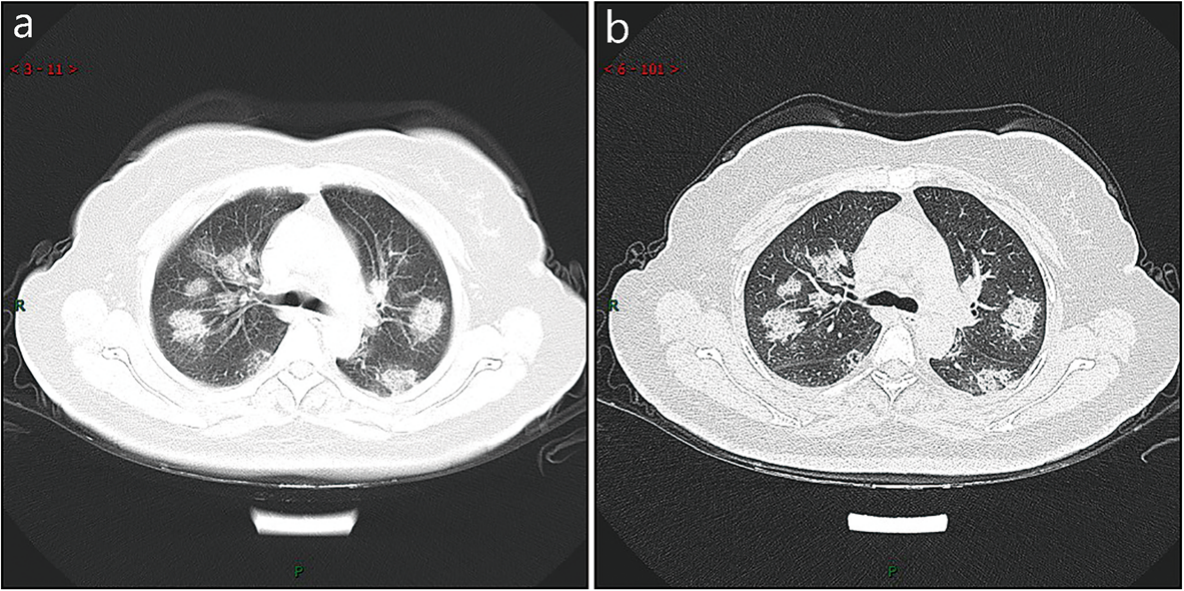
CT imaging of rapid progression stage. A 50 years old female with anorexia, fatigue, muscle soreness, nasal congestion and runny nose for 1 week, sore and itching throat for 2 days. Laboratory test: increased erythrocyte sedimentation rate (25 mm/h), normal white blood cells (4.08 × 10^9^/L), decreased lymphocytes (0.96 × 10^9^/ L), increased C-reaction protein (60.8 mg/L). Imaging examination: a (thin layer CT) and b (high-resolution CT) showed multiplepatchy and light consolidation in both lungs and grid-like thickness of interlobular septa
